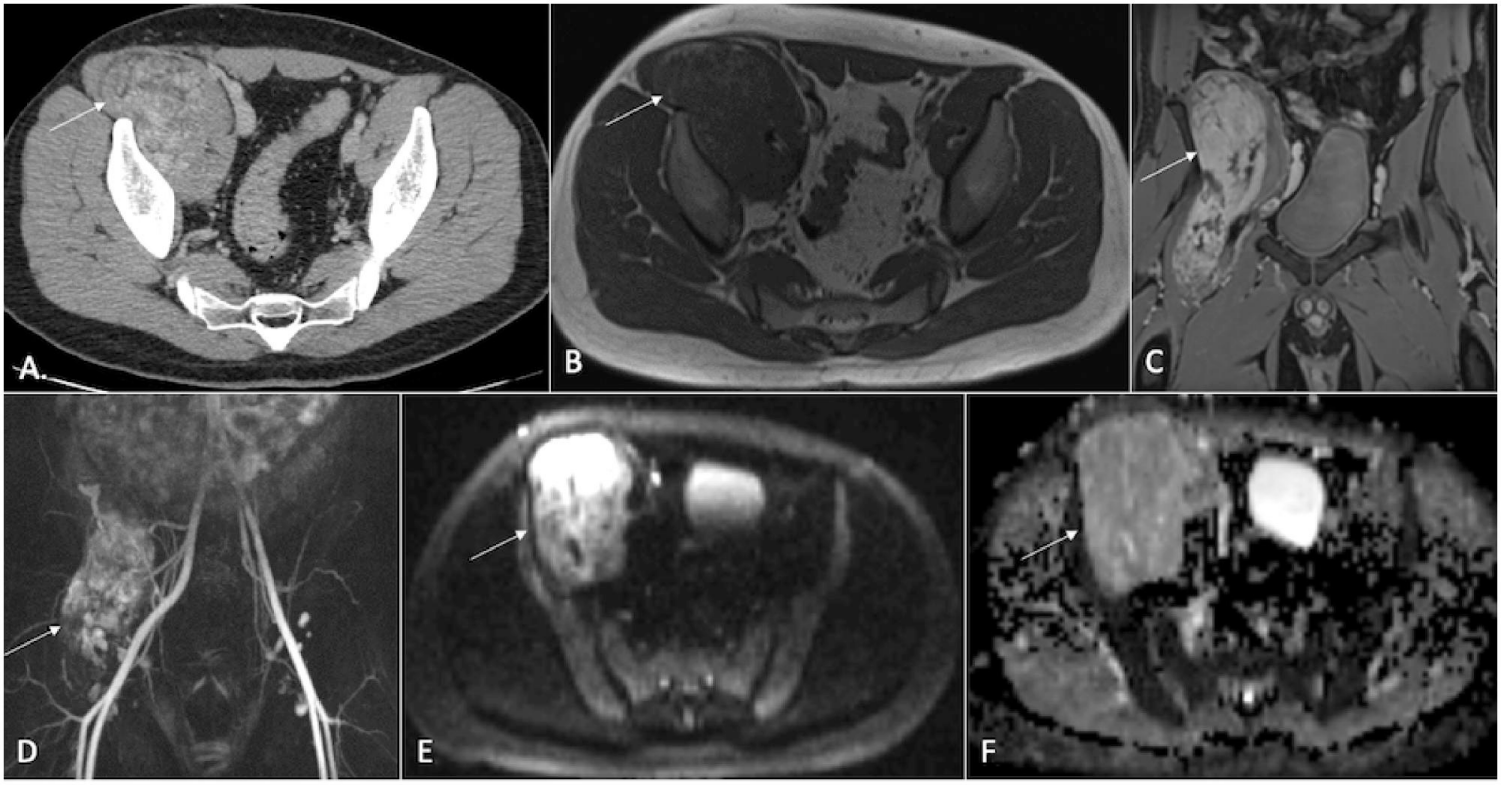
A 27-year-old male has an intramuscular hemangioma located in the right iliopsoas muscle. Lesions is marked by arrow. A post-contrast CT axial section reveals heterogeneous enhancement within the lesion along with fatty streaks. The T1-weighted image displays a hypointense mass with hyperintense streaks suggestive of fat. The post-contrast T1-weighted sequence shows heterogeneous enhancement. The TWIST (Time-resolved Imaging with Stochastic Trajectories) (D) indicates hypervascularity. DWI (E) demonstrates hyperintensity with no corresponding defect on the ADC map (F), suggesting a benign nature.
